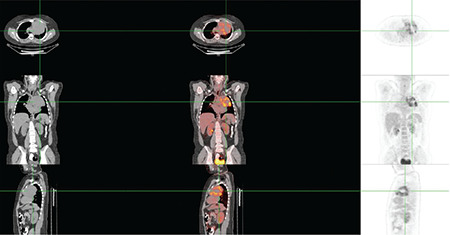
Heterogeneous uptake with increased ^18^F-FDG accumulation in large anterior mediastinal mass, which was immature teratoma, as shown by the transaxial, coronal, and sagittal plane CT, fusion, and PET images ^18^F-FDG: ^18^F-fluorodeoxyglucose, PET: Positron emission tomography, CT: Computed tomography
